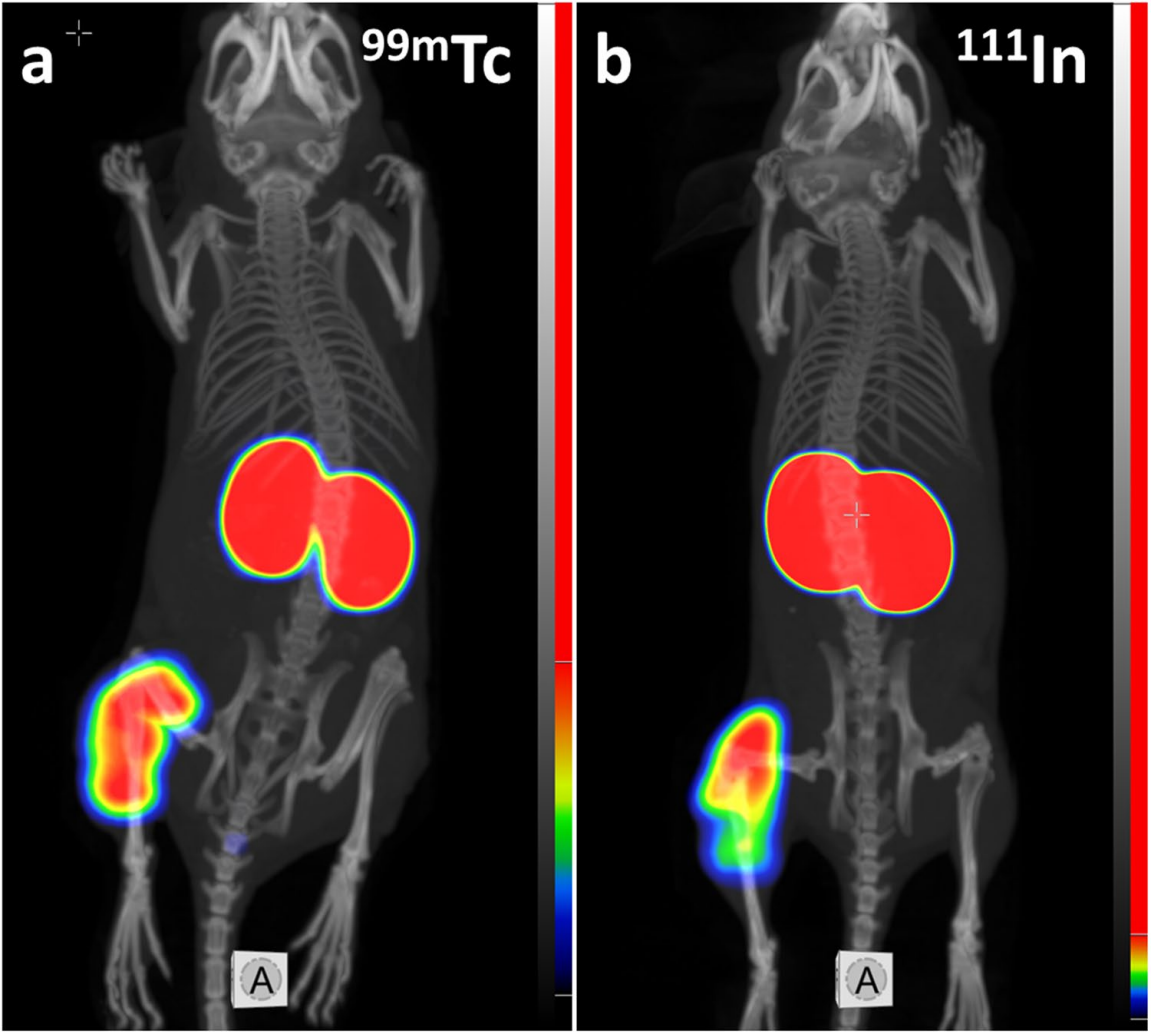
MicroSPECT/CT imaging of HER2-expressing SKOV3 xenografts (left hind legs) at 4 h after injection of ^99m^Tc-DEAVDANS-ADAPT6-GSSC (a) and ^111^In-DEAVDANS-ADAPT6-GSSC-DOTA (b). Color scales were adjusted to provide clear visualization of tumors.
